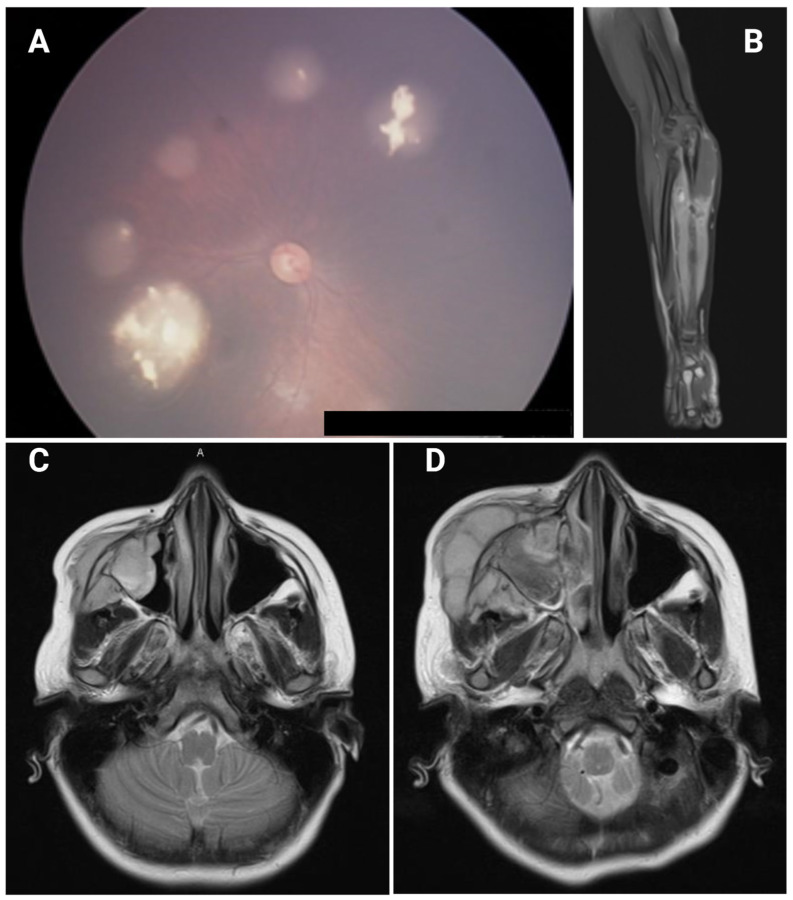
(A) Multifocal RB in the right eye with calcium content in its interior. (B) MRI of the left forearm showing a hyperintense lesion in the cubitus with disruption of bone cortex. (C) Head MRI at time of diagnosis showing a giant lobulated tumor in the right maxilla with extension to the ipsilateral maxillary sinus space. (D) Head MRI three months after diagnosis showing a giant lobulated tumor in the right maxilla with extension to the ipsilateral maxillary sinus space.
